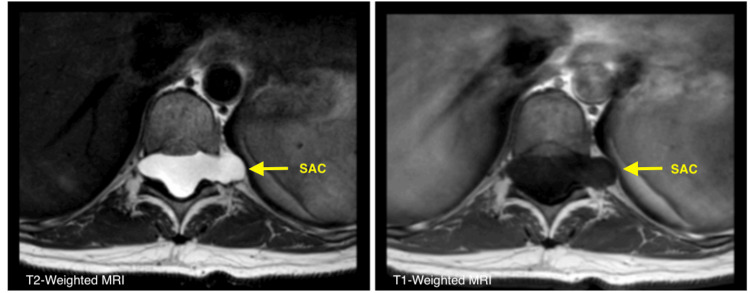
Preoperative axial T2-weighted MRI showing bilateral extension of the cystic lesion into the neural foramina, with greater involvement on the left side The lesion is isointense to cerebrospinal fluid and compresses adjacent neural structures.
